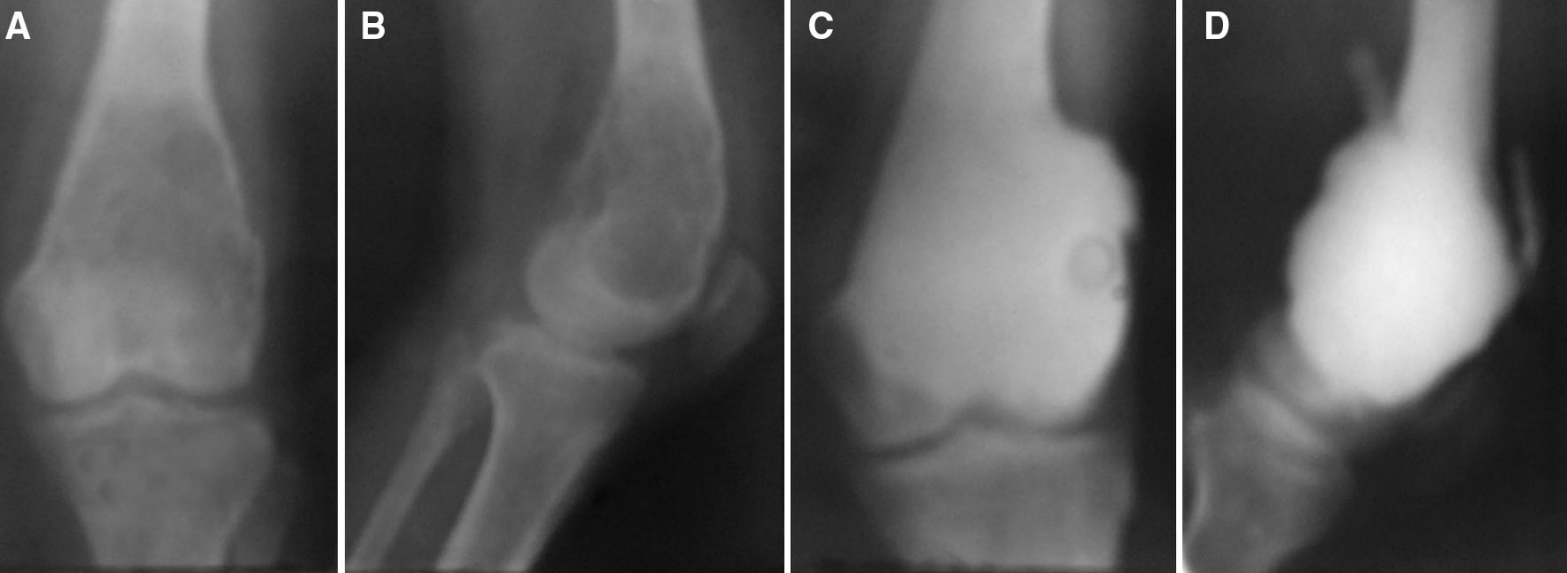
Antero-posterior and lateral radiographs of a 33-year-old female showing an extensive lesion in the distal femur with a pathological fracture (a, b). Follow-up at 8 years after after curettage and cementation (c, d). The patient had good range of motion at the knee
